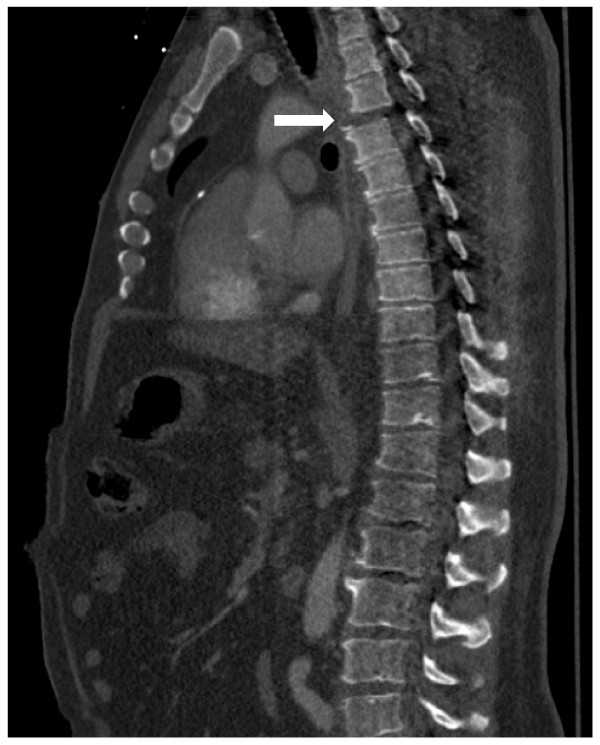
Lateral CT scan of thoracic spine demonstrates T3/4 fracture dislocation (white arrow).
